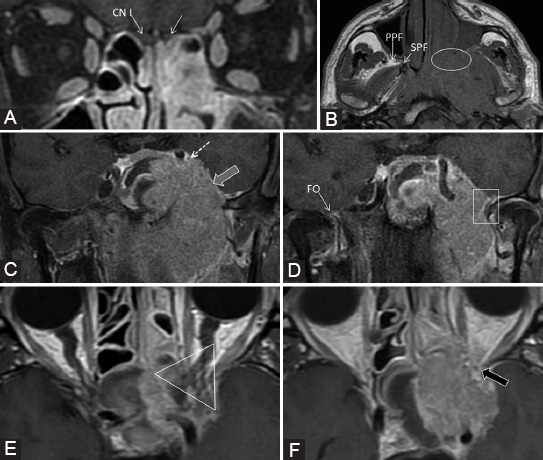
The left rhinopharyngeal squamous cell carcinoma with locoregional and skull base invasion. Coronal T1-weighted fat-saturated images (A, C, D); axial T1-weighted (B) and axial contrast-enhanced T1-weighted FSE (E, F) images. There is CE at the level of the left CN I (white arrow) which is invaded directly by the tumor (T). The PPF and sphenopalatine fissure (SPF) fat effacement (ellipse) confirms the involvement of V2 branch. There is enlargement and CE of the left Meckel’s cave (gray arrow) and cavernous sinus (dashed arrow), indicating the PNS along the CNs III, IV, V1, V2, and VI. Notice the foramen ovale invasion (CN V3, rectangle) compared to the right side (FO). The orbital apex together with the CN II is included in the tumor (triangle) and there is extension of pathologic tissue in the left superior orbital fissure (black arrow). Observe the slightly grayer appearance of CE (“evil gray”) on the non-fat-saturated sequences compared to the bright signal of fat. Notice the corresponding contralateral normal structures marked on the images.
